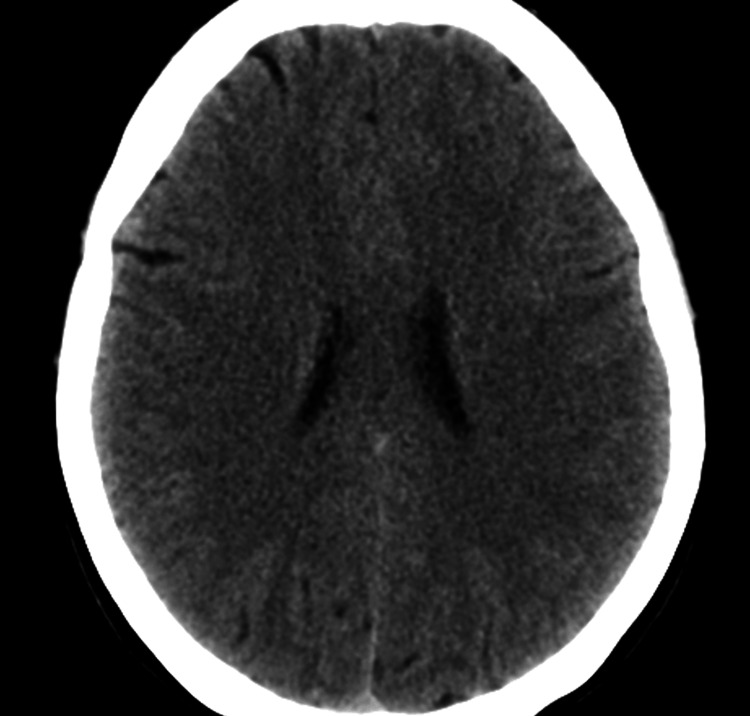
Normal non-contrast CT of the brain Axial non-contrast computed tomography (CT) image of the brain demonstrates no acute intracranial abnormalities. There is no evidence of intracranial hemorrhage, mass lesion, hydrocephalus, or midline shift. The ventricles and sulci are normal in size and configuration.
